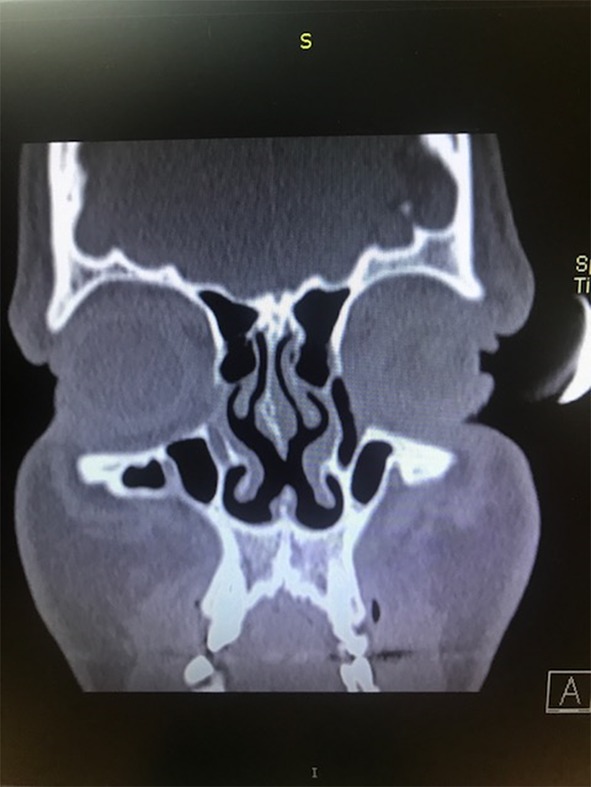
The computed tomography scan of the septal perforation in the same patient
